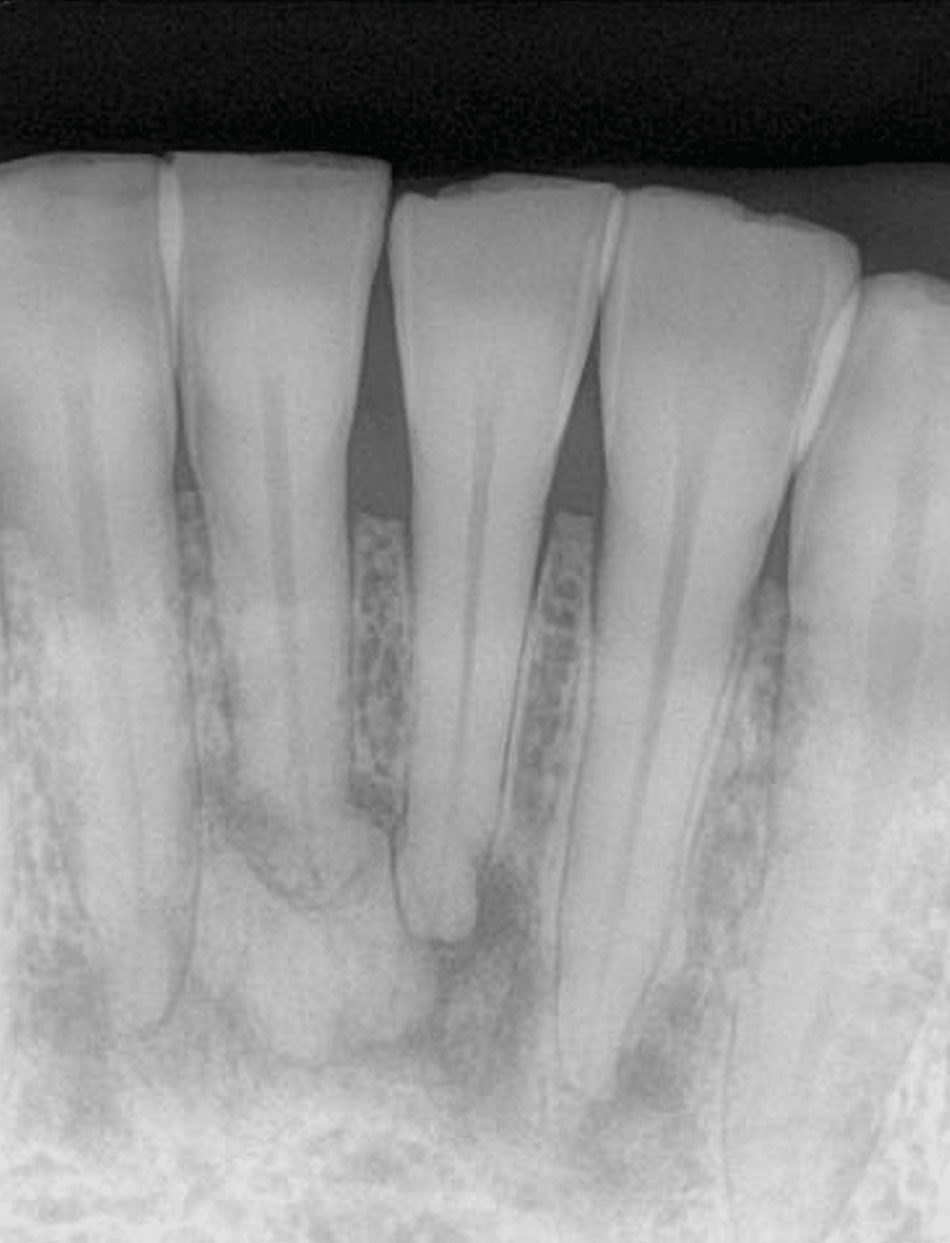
Mixed radiolucency and radiopacity periapical lesion.
